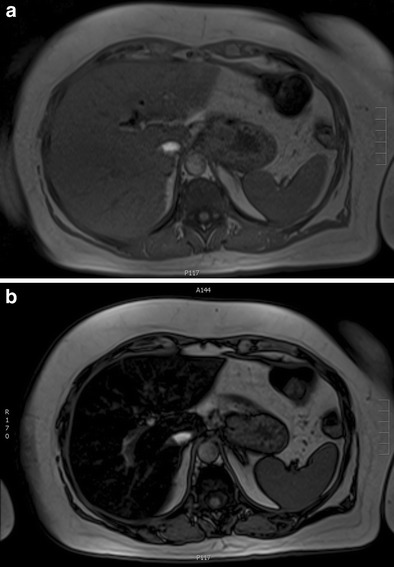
A 60-year-old female with colorectal cancer currently being treated with irinotecan. a, b Axial T1-weighted MR images in and out of phase, respectively, show significant signal drop in the liver consistent with steatosis
